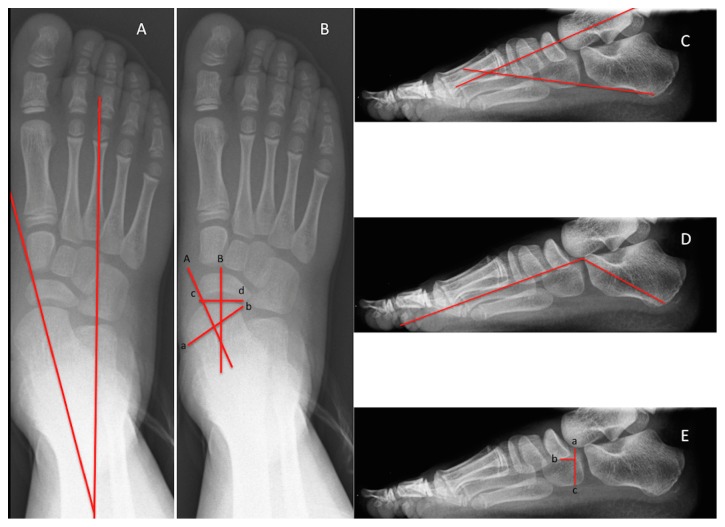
The graphical representation of angles measures used in the study. A: DP talocalcaneal angle. B: talonavicular coverage angle. Points a and b = medial and lateral edges (resp.) of the articular surface of the talus bone. Points c and d = medial and lateral edges (resp.) of the articular surface of the navicular bone. Line A is perpendicular to ab, and line B is perpendicular to cd. Talonavicular coverage is the angle created by the intersection of lines A and B. C: talocalcaneal angle in lateral radiography. D: Moreau-Costa-Bartani angle. E: naviculocuboid overlap. Points a and b = superior and inferior margins (resp.) of the cuboids. Point c = inferior margin of the navicular. Naviculocuboid overlap = ac/ab X 100.
